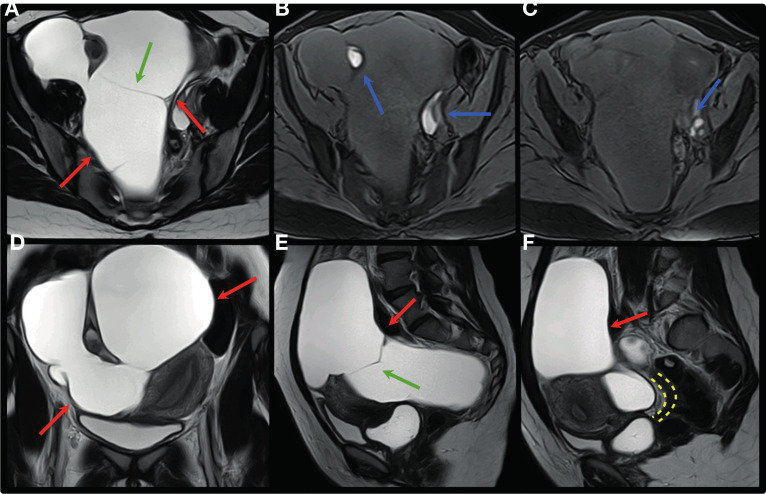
Axial, coronal and sagittal T2W (A, D, E, F) and axial T1W MR images with fat saturation (B,C) show large cyst of peritoneal inclusion occupying practically the entire pelvic cavity (red arrows), with some fine septations (green arrows), bilateral ovarian endometriomas (blue arrows) and deep endometriosis infiltrating the anterior rectosigmoid wall (yellow interrupted line).
